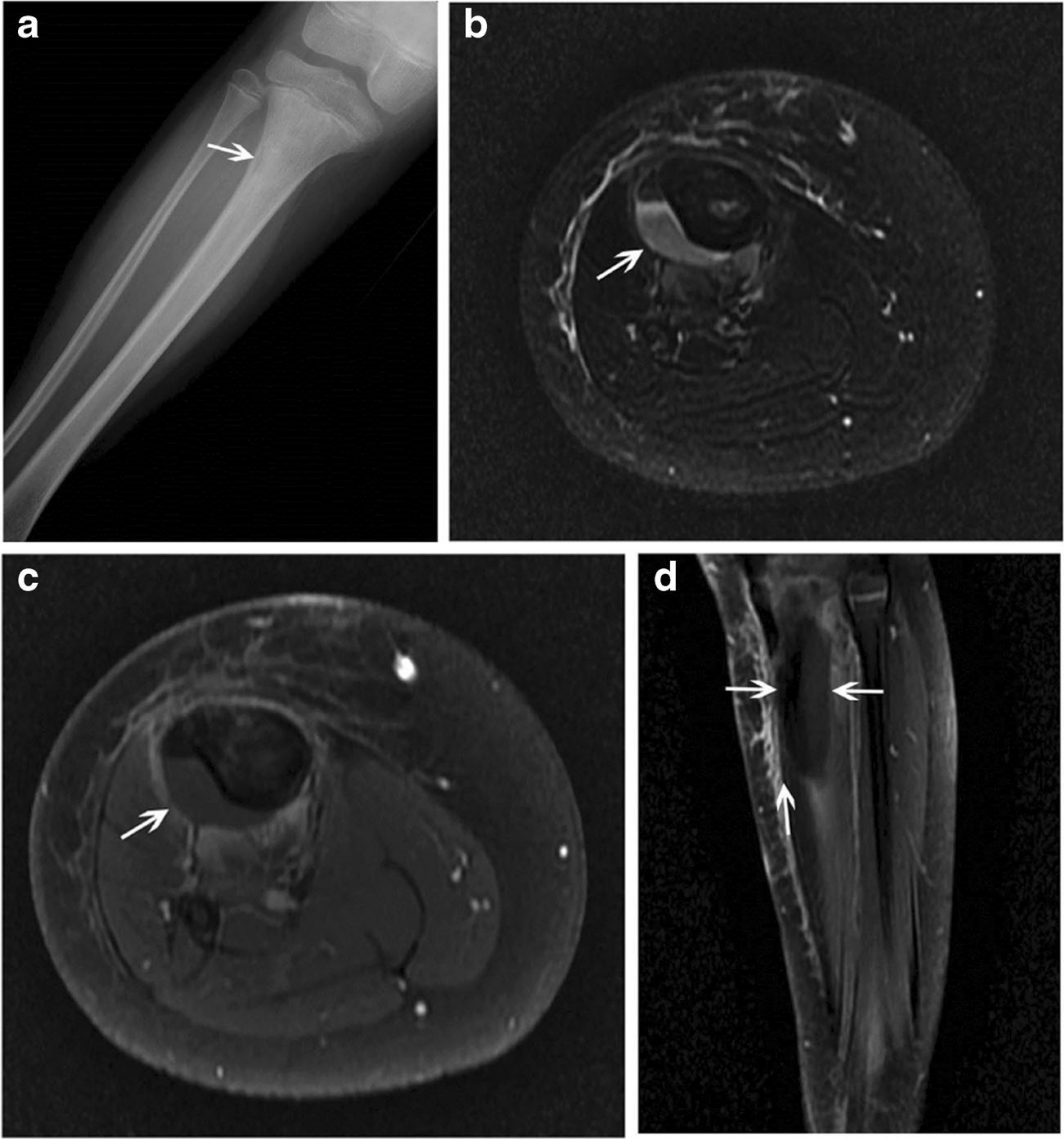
Septic cortical osteitis with subperiosteal abscess formation in a 7-year-old girl. Antero-posterior radiograph of the left tibia a shows an ill-defined lucency at the lateral aspect of the proximal tibial metaphysis (arrow). Axial  STIR image b reveals a crescentic, hyperintense fluid collection adjacent to the proximal tibia at this level. This collection is hypointense with peripheral enhancement as seen on the post-contrast axial c and sagittal d images. Enhancement can also be seen through surrounding soft tissuesDuring the subacute phase of an evolving bony infection, the transition zone between normal and abnormal bone becomes sharper as the process becomes more localized [21].-Intracortical Brodie’s abscess:Brodie’s abscess is a manifestation of subacute osteomyelitis, and though usually metaphyseal/medullary in location, can rarely be intracortical. When intracortical in location, it can present radiographically as an irregular lytic abscess cavity within the bony cortex with associated solid periosteal reaction [1].-Chronic recurrent multifocal osteomyelitis (CRMO):CRMO is a non-bacterial, auto-inflammatory disease characterized by recurrent flares of inflammatory bone pain related to presence of multiple foci of aseptic osteomyelitis [22]. Children and adolescents comprise the main affected demographic with the mean age at manifestation determined to be 11.4 years on a German incidence surveillance study (Fig. 14) [23].
